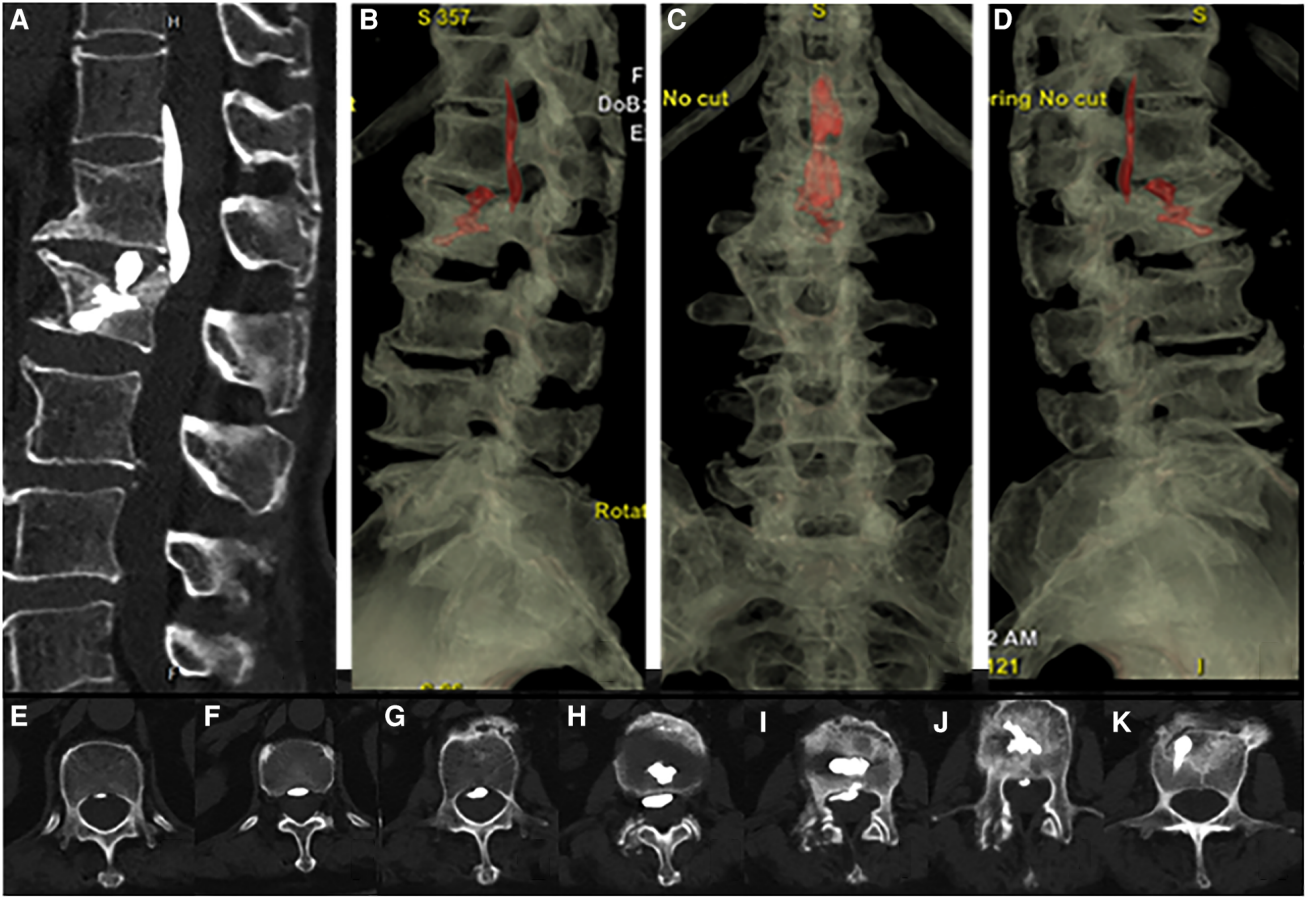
Postoperative CT scan 3D reconstruction showed bone cement infiltrating the epidural space of the spinal canal and distributed along the posterior longitudinal ligament from T12 to L2 (A–D) and the filling of bone cement in the posterior spinal canal of the L2 vertebral body(E–K).
